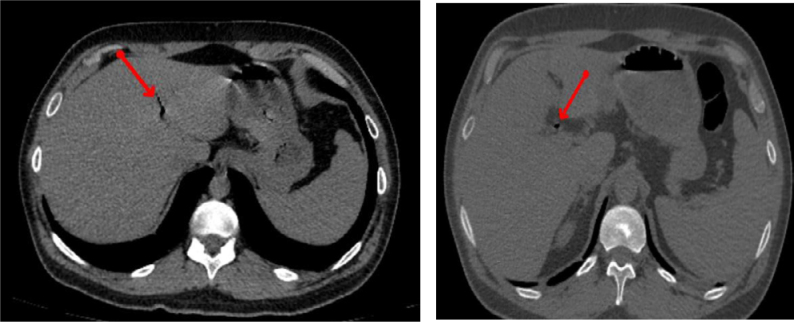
Abdominal computed tomography scans showing aerobilia (arrows — left and right).
